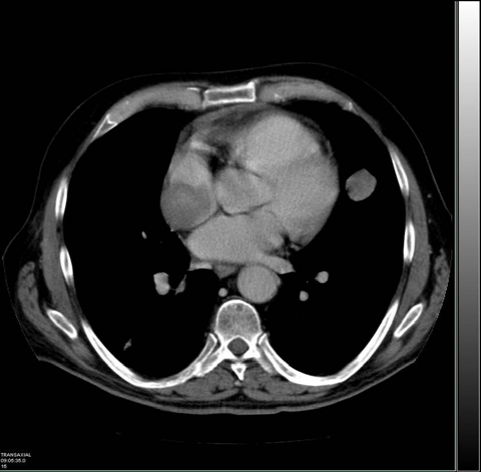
Second CT scan showing a right-atrial mass, measuring 5.7 cm in diameter together with pulmonary lesions.
